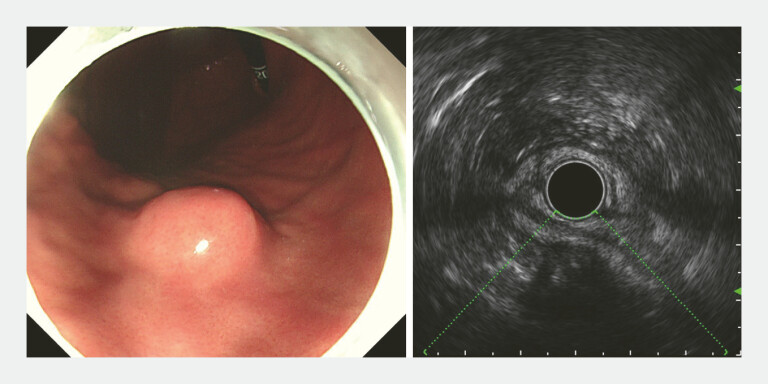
Endoscopic and EUS images. Endoscopy revealed a submucosal tumor on the greater curvature of the gastric body. EUS showed the hypoechoic lesion originated from the muscularis propria layer. EUS, endoscopic ultrasound.
